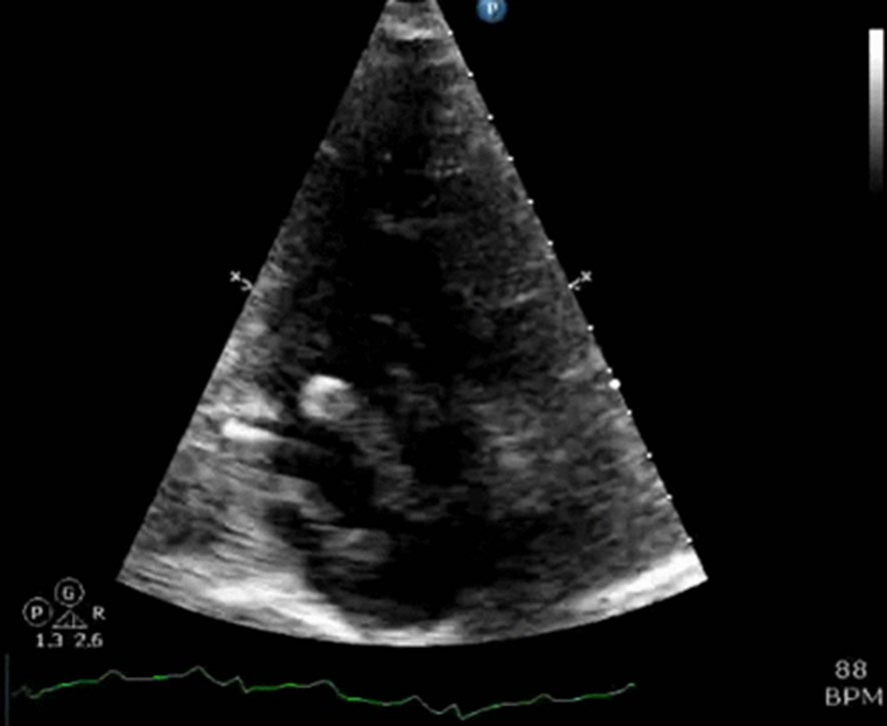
Apical 4-chamber view with a large mobile thrombus in the right atrium crossing into the right ventricle. There is flattening of the interventricular septum consistent with right ventricular pressure overload. Mc Connell’s sign is present
